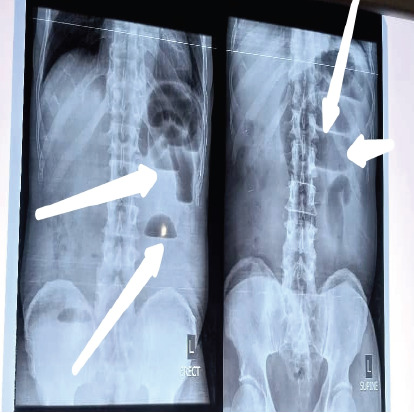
Plain abdominopelvic radiograph showing multiple air-fluid level as indicated by the white arrow
